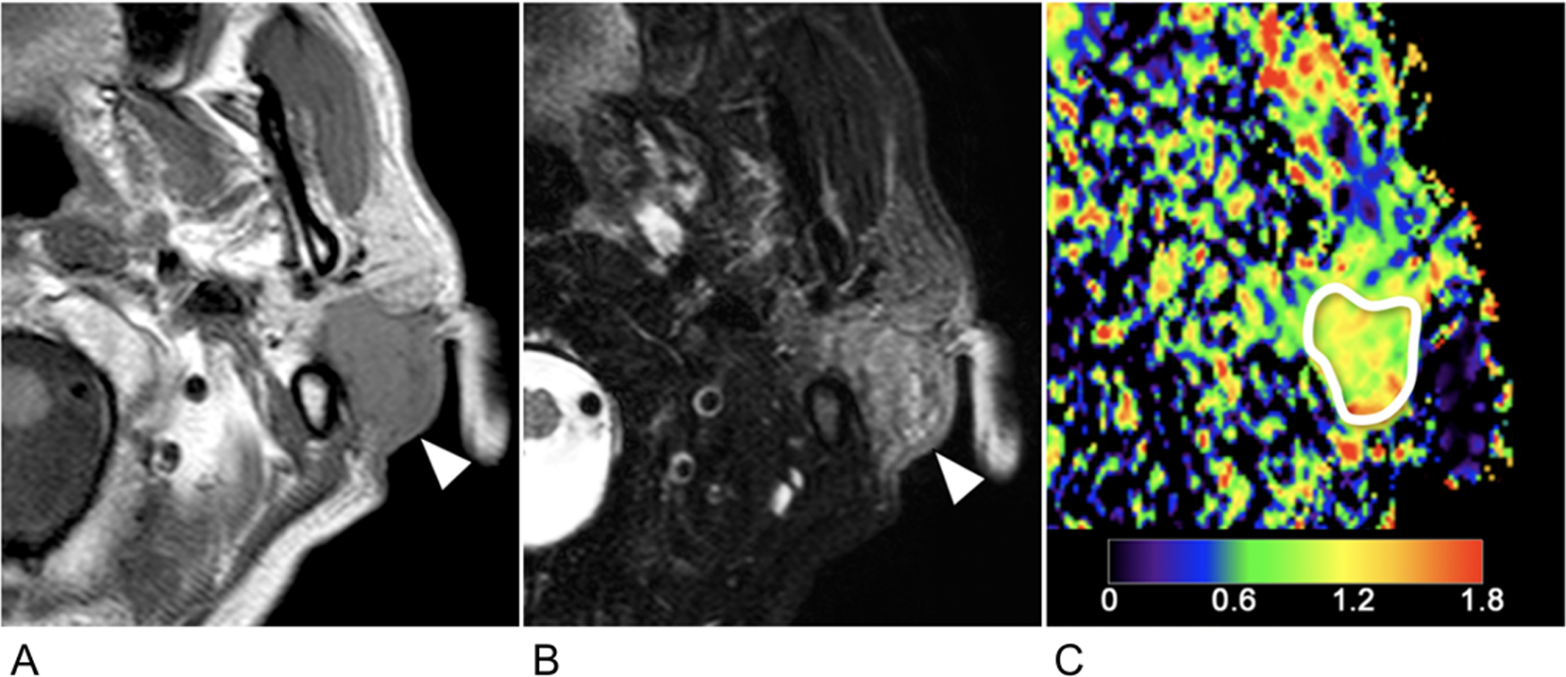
67-year-old man with mucoepidermoid carcinoma (T4N0M0). A, Axial T1-weighted MR image shows homogeneous and invasive cancer arising in left parotid gland (arrowhead). B, Axial fat-suppressed T2-weighted MR image shows heterogeneous parenchyma (arrowhead). C, Axial, color ADC map. White demarcation indicates an ROI manually placed within the tumor area for ADC measurement. Color scale bar indicates ADC levels (0–1.8 × 10^−3^ mm^2^/s). He had survived beyond 3 years (77 months) after surgical excision of primary cancer.
